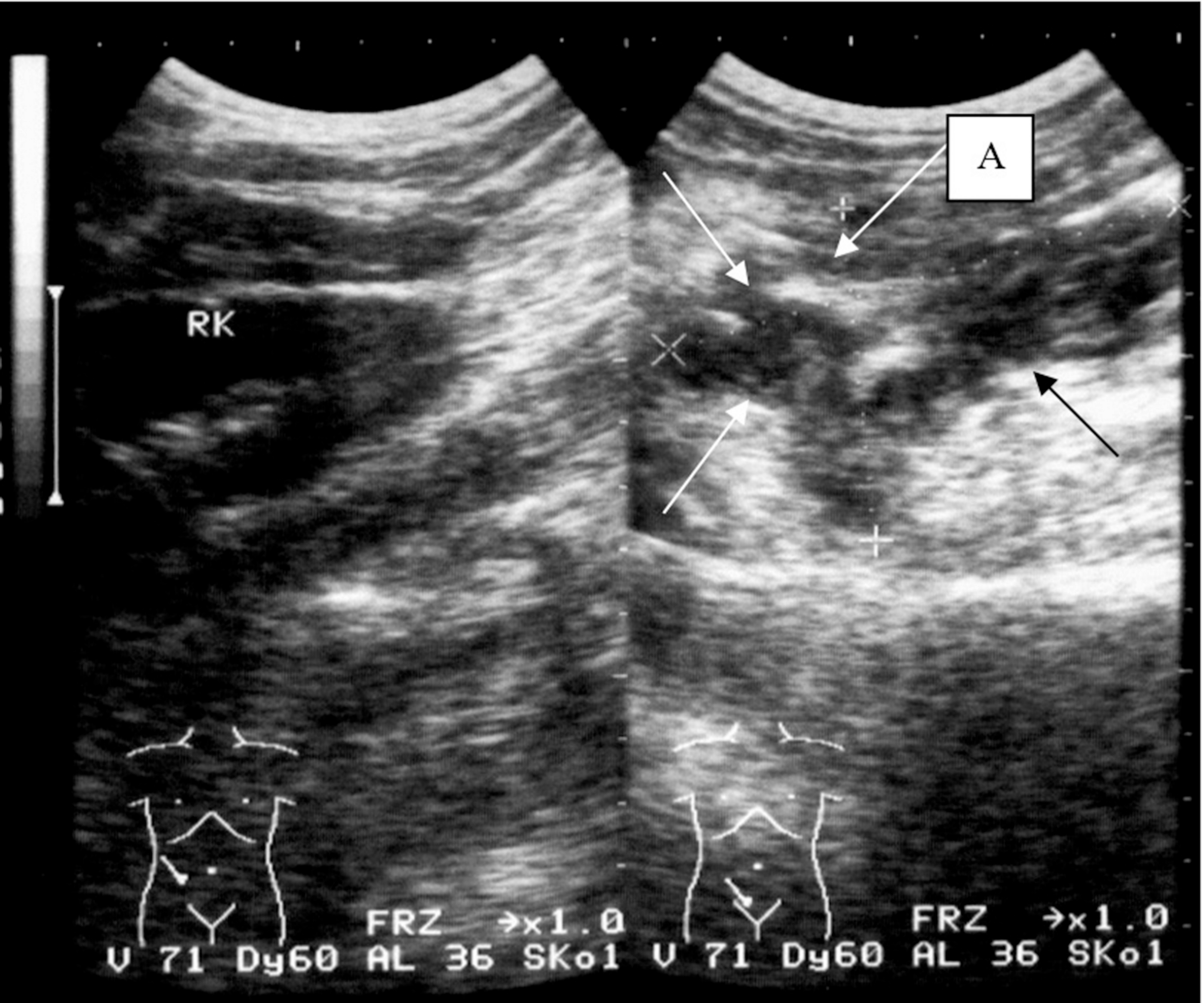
X-ray image of the abdominal abscess in the right iliac region (arrows) and the ileocecal angle of the intestine (A).
